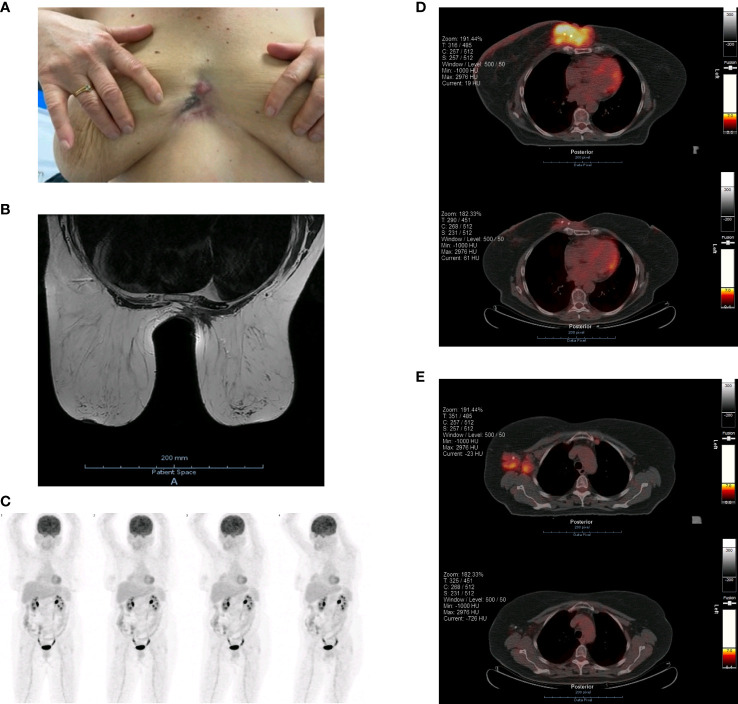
(A) Clinical response following 6 months of systemic therapy. (B) Breast MRI following 6 months of systemic therapy. (C) Whole body on PET/CT with 18F-FDG following 6 months of systemic therapy. (D) Right breast lesion on PET/CT with 18F-FDG prior to (upper image) and following 6 months of systemic therapy (lower image). (E) Lymph node involvement on PET/CT with 18F-FDG prior to (upper image) and following 6 months of systemic therapy (lower image).
